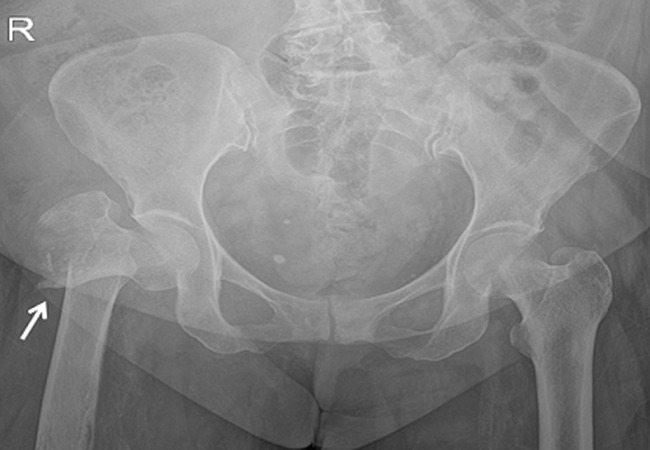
preoperative X-ray of pelvis showing displaced intertrochanteric fracture right femur
